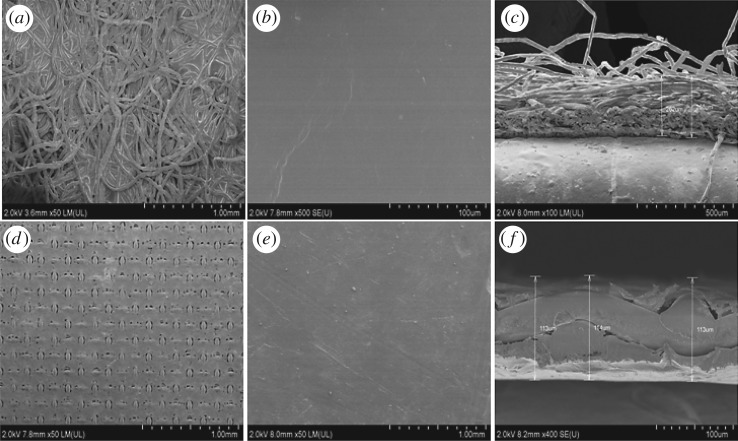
SEM: (*a*) support layer of CTA-NW; (*b*) active layer of CTA-NW; (*c*) cross-section of CTA-NW; (*d*) support layer of TFC-ES; (*e*) active layer of TFC-ES and (*f*) cross-section of TFC-ES.
